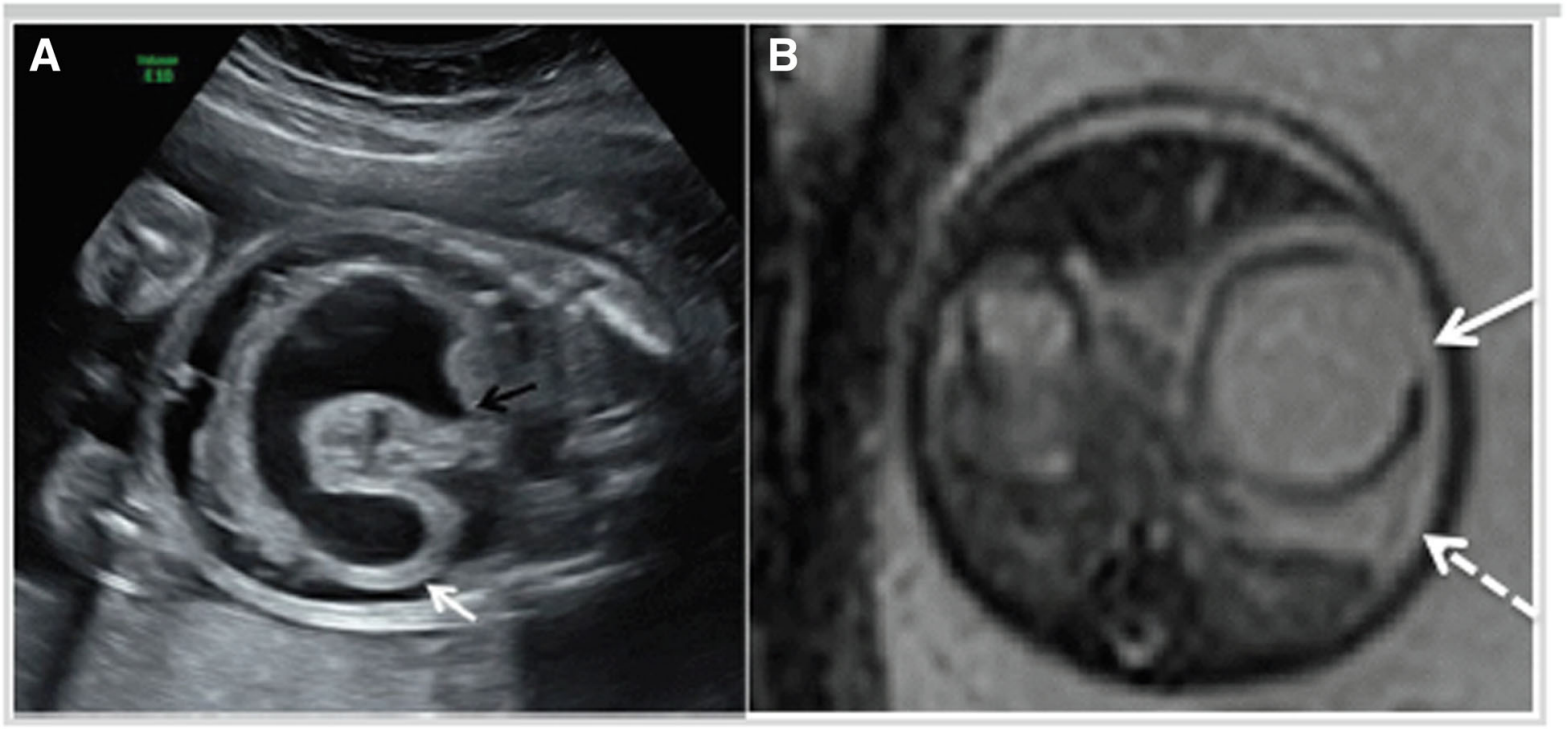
a Transverse ultrasound image of twin B showing the dilated and thick-walled stomach with a “cut-off” at the second portion of the duodenum (*white arrow*). *Black arrow* shows the area of fluctuation of the gastroesophageal junction. b Axial T2-weighted magnetic resonance image of the twin pregnancy showing the dilated fluid-filled stomach with evidence of wall rupture (*white arrow*) and subsequence ascites (*dashed arrow*)
